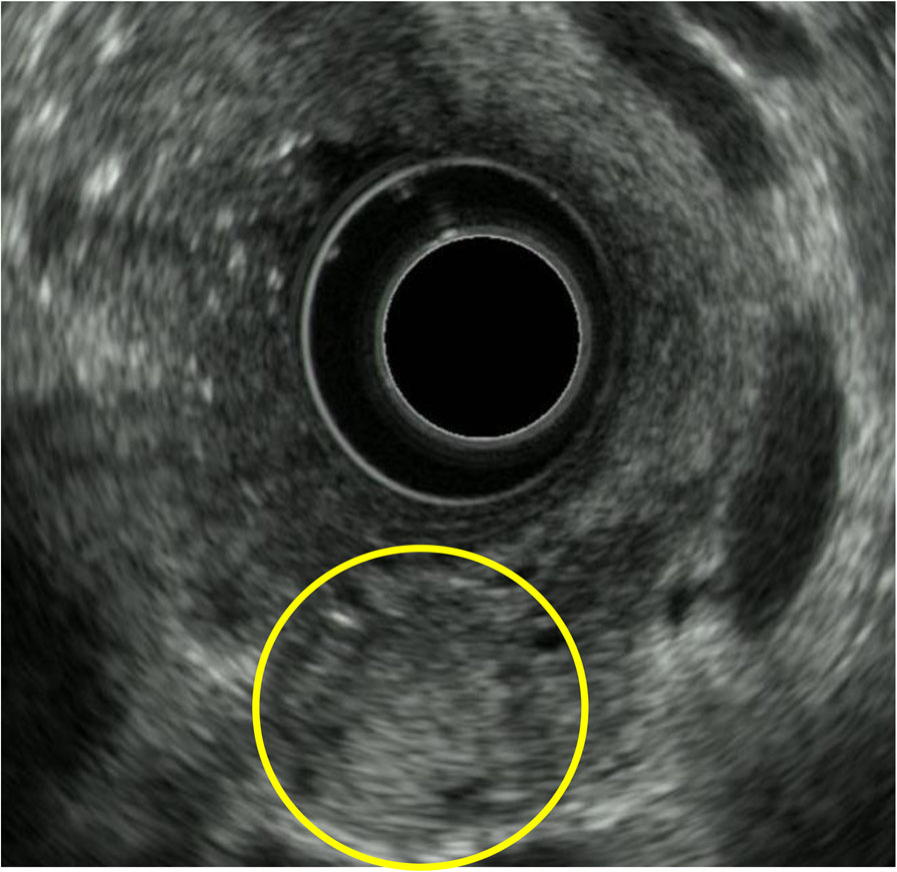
Endoscopic ultrasound sonography showing the high echoic tumor at the pancreatic head
